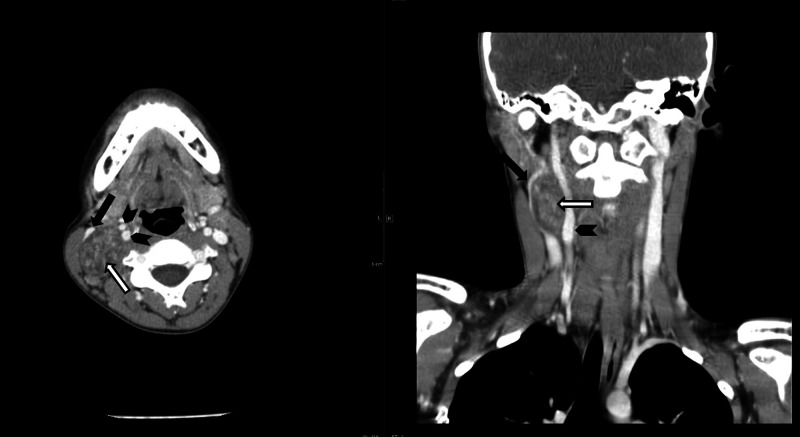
Axial and coronal contrast enhanced CT showing vagal schwannoma (white arrow) next to carotid artery (arrowhead) and compressing internal jugular vein (black arrow). In the axial image, distinction can be seen between the internal and external carotid arteries, supporting the tumor's suspected location within the carotid sheath at the level of the carotid bifurcation.
